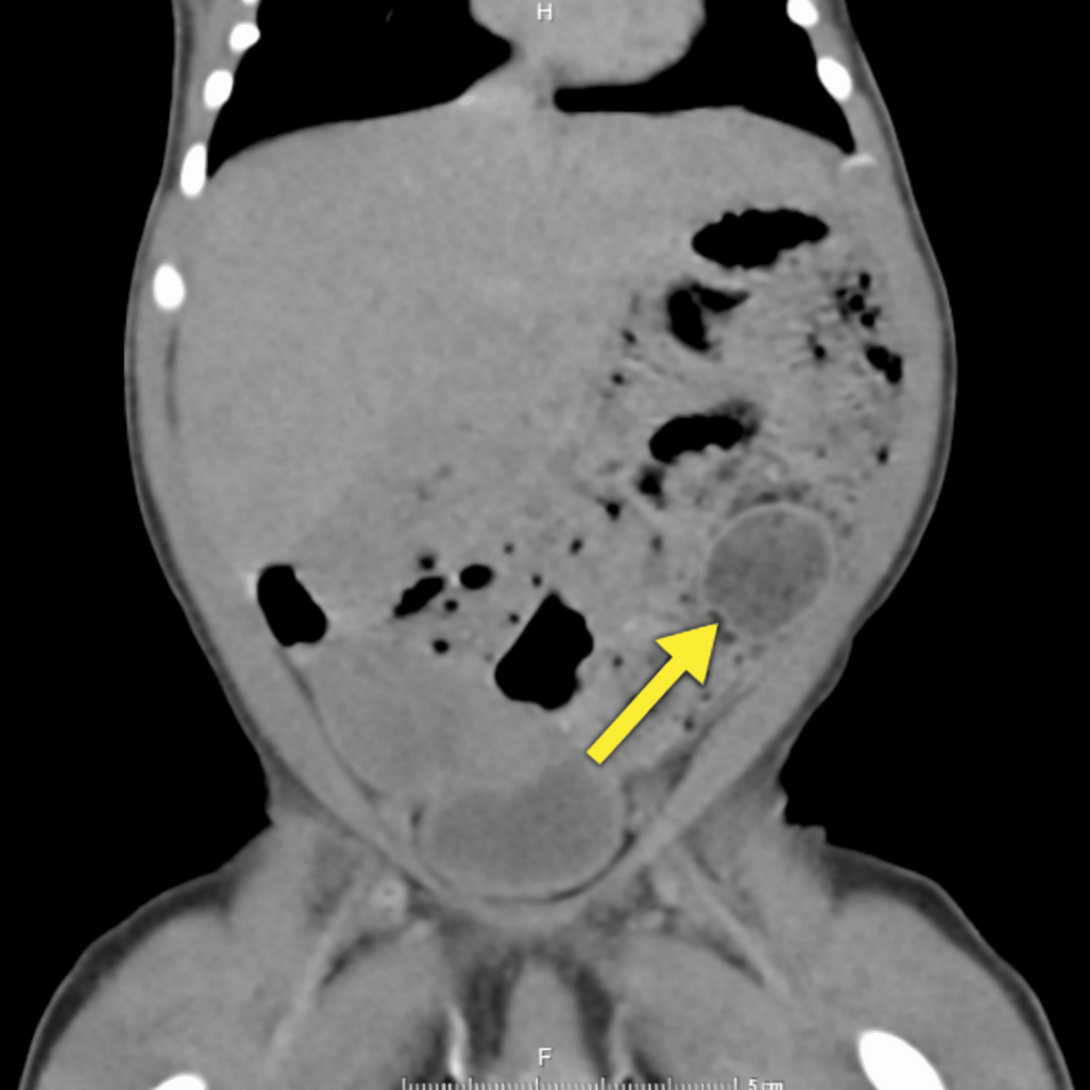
CT scan findings Multislice scan of the abdomen and pelvis demonstrating a well-defined mass in the left iliac fossa measuring 29.2 × 20.8 × 26.2 mm (arrow) CT: computed tomography
